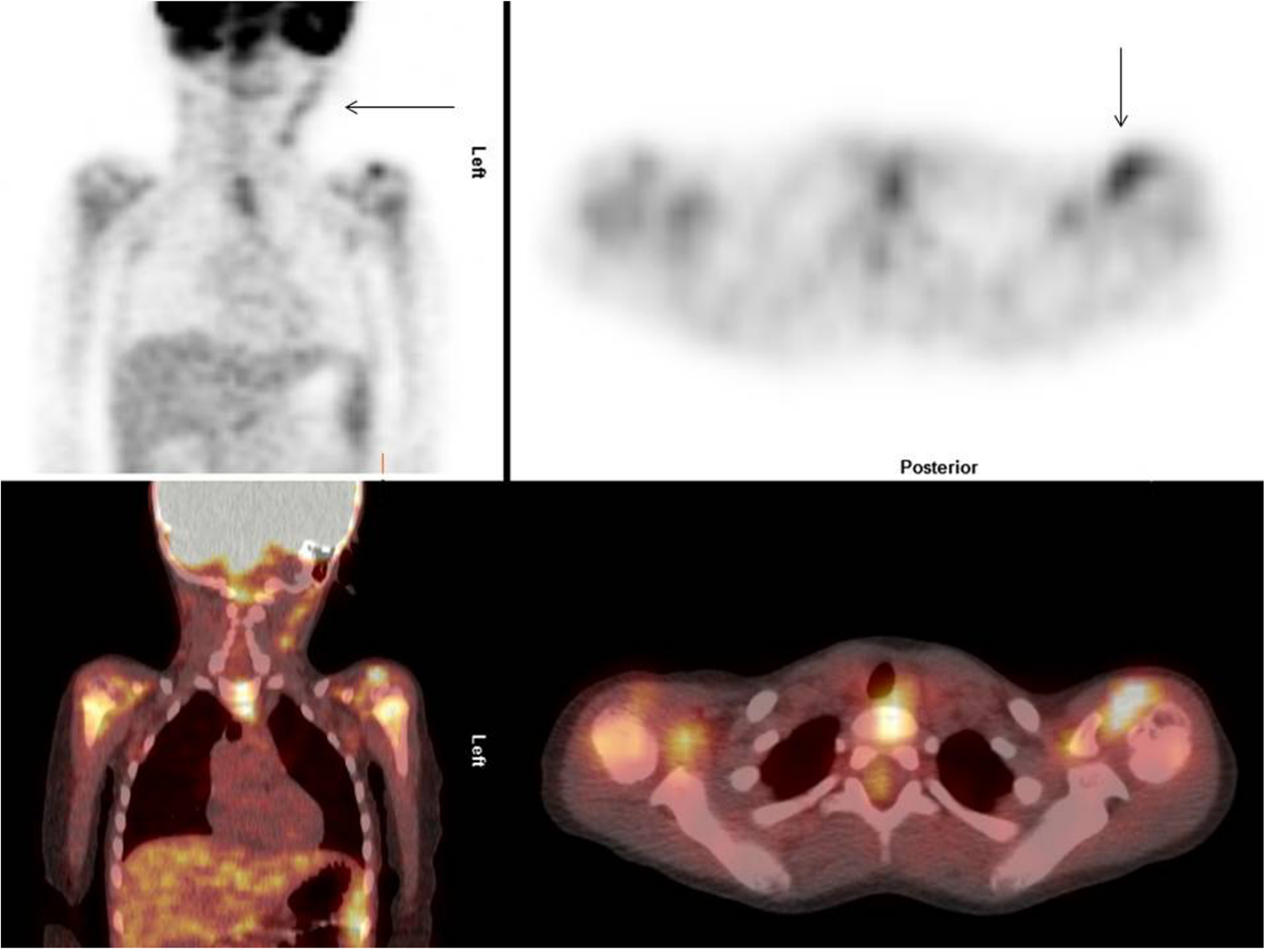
Detailed images of fludeoxyglucose positron emission tomography-computed tomography scan showing metabolically active cervical lymph nodes and increased fludeoxyglucose uptake in the left shoulder joint (*arrows*)
